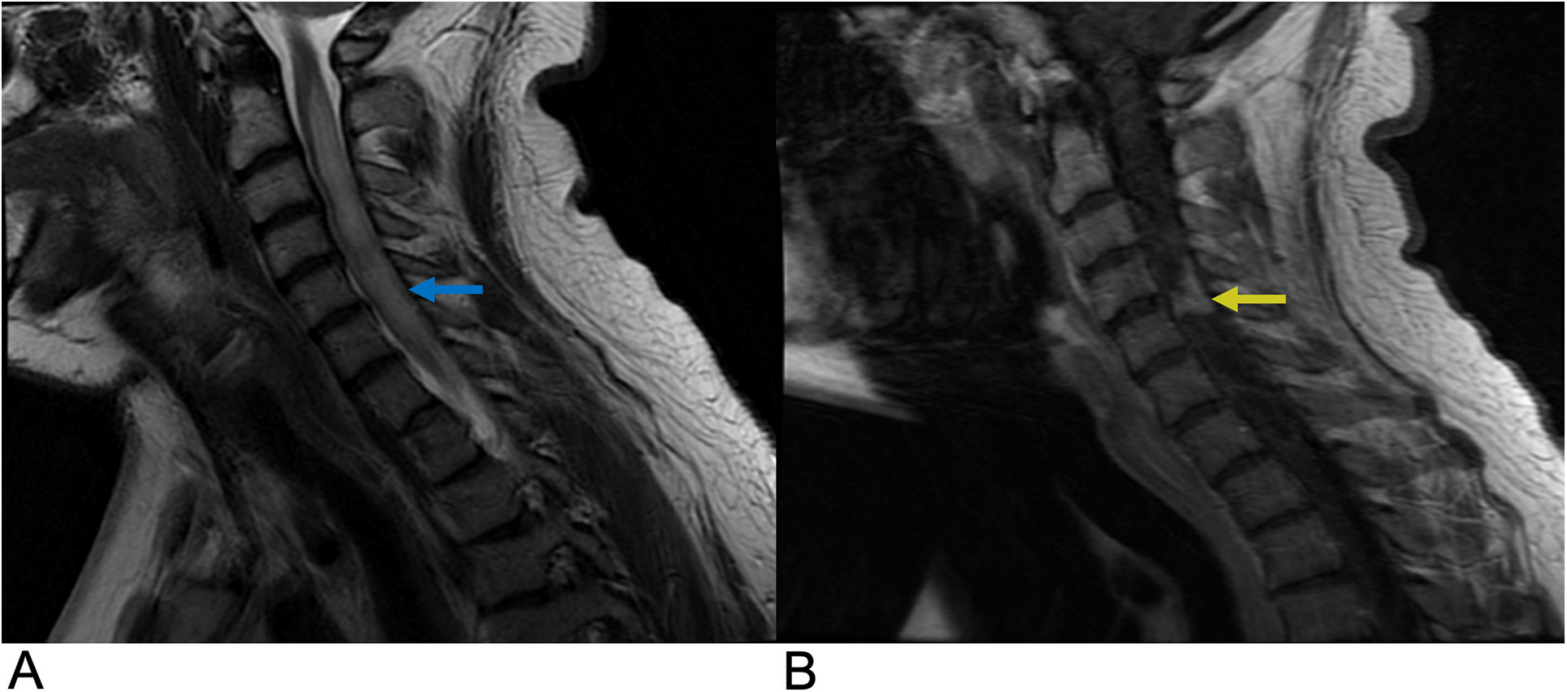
Transverse myelitis. Sagittal T2 (A) and sagittal T1 + C (B) MRI demonstrating a long segment of T2 (blue arrow) and T1C hyperintense signals (yellow arrow) occupying greater than two-thirds of the cross-sectional area of the cord representing transverse myelitis.
